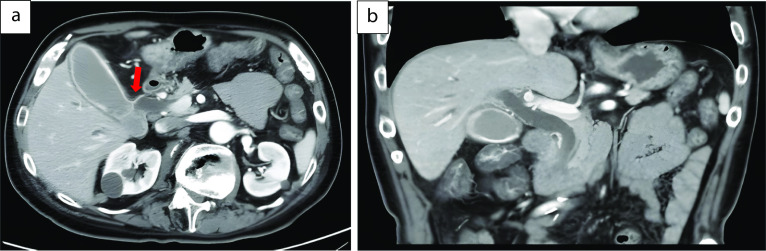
Abdominal contrast-enhanced CT. Axial view (a) and coronal view (b) reveal diffuse thickening of the gallbladder wall and dilatation of the common bile duct, but no evidence of extravasation within the gallbladder or bile duct. There are no obvious findings of gallstones, cholecystitis, tumors, or aneurysms. The red arrow indicates cystic artery. CT, computed tomography
